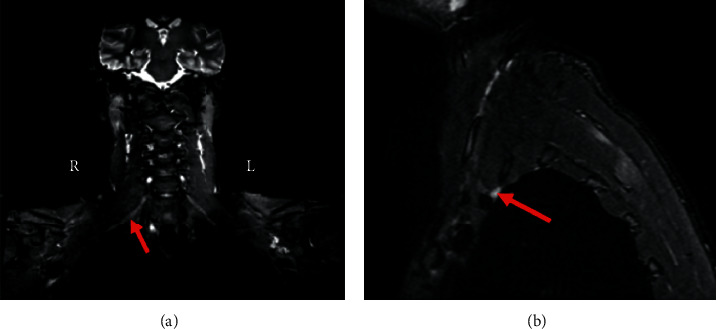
Chest MRI of the patient with inflammatory brachial plexopathy. (a) Coronal chest and (b) sagittal chest, T2 weighted and fat-suppressed MRI images without contrast show elevated T2 signaling indicating subtle thickening in the right brachial plexus structure at the time of diagnosis.
